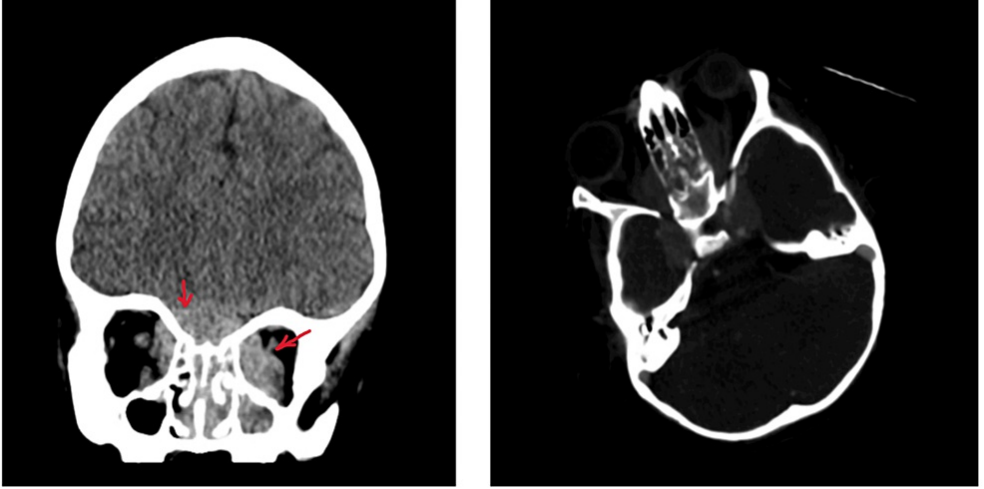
CT Brain showed a large enhancing mass in the base of the skull measuring 6.1 x 4.1 cm involving both anteromedial temporal and the inferior frontal lobes, cavernous sinus bilaterally, nasal sinuses and extending to the orbits bilaterally with mass effect on the optic nerve.
